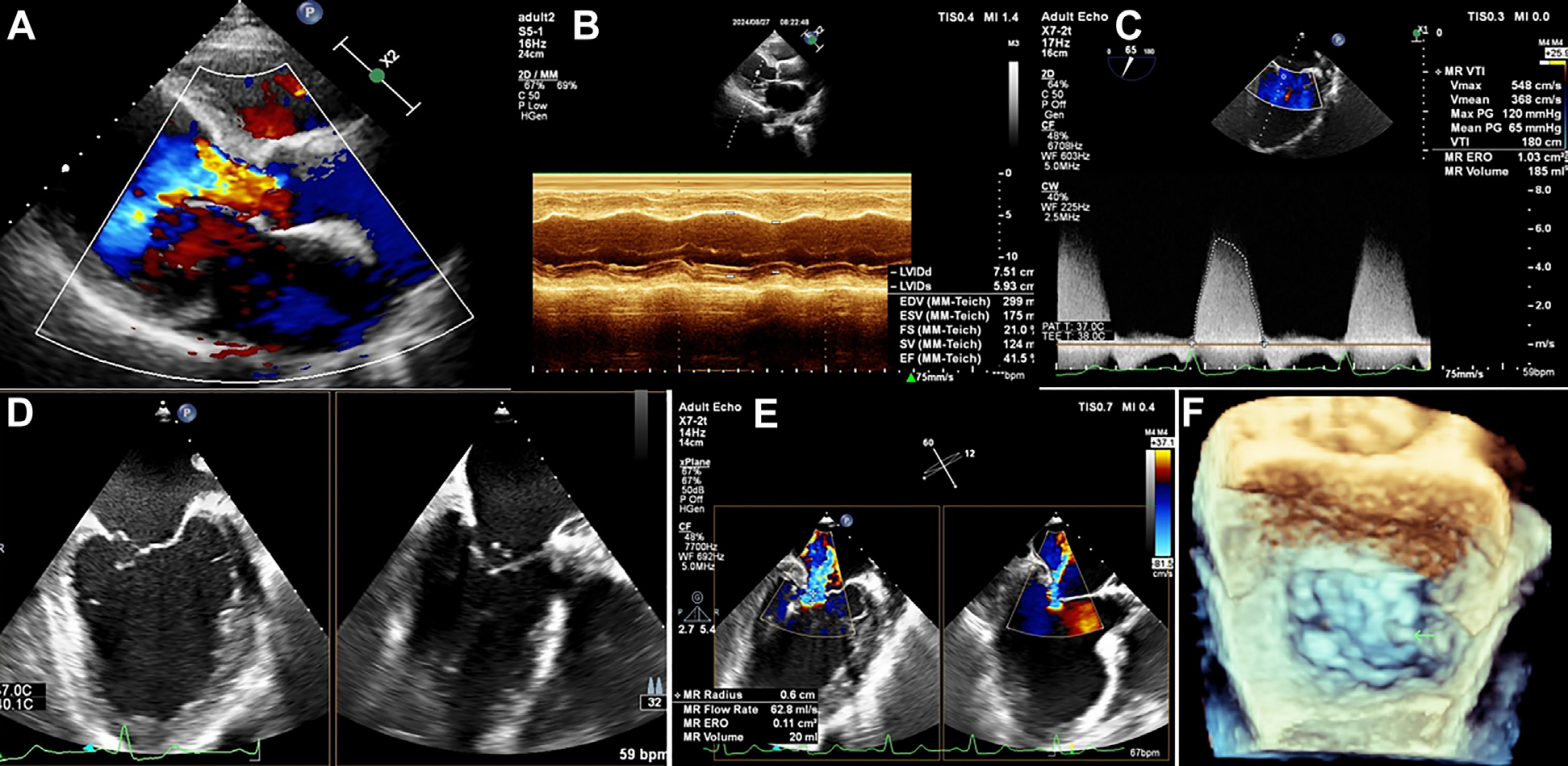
Evaluation of Aortic Regurgitation and MR by Thoracic and Transesophageal Echocardiography (A) Aortic regurgitation; vena contracta = 12 mm. (B) Left ventricular dilation; left ventricular ejection fraction = 41.5%. (C) Mitral regurgitation (MR); effective regurgitant orifice (ERO) area = 1.03 cm^2^. (D) Biplane images in bicommissural and left ventricular outflow tract views by transesophageal echocardiography. (E) Effective regurgitant orifice area and proximal isovelocity surface area radius. (F) 3-dimensional en face surgery view; the arrow shows ruptured chordae tendineae. 2D = 2-dimensional; bpm = beats/min; EDV = end-diastolic volume; EF = ejection fraction; ESV = end-systolic volume; LVIDd = left ventricular internal diameter in diastole; LVIDs = left ventricular internal diameter in systole; max = maximum; PG = pressure gradient; SV = stroke volume; V = velocity; VTI = velocity time integral.
